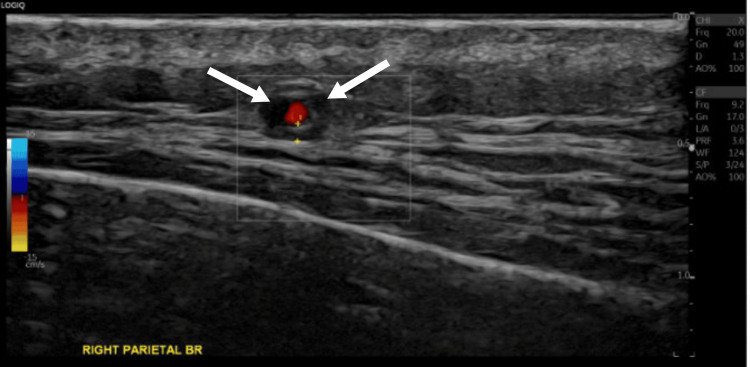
Temporal artery color Doppler ultrasound (right parietal branch) Ultrasound image showing marked hypoechoic "halo sign" (arrows) and circumferential wall thickening
